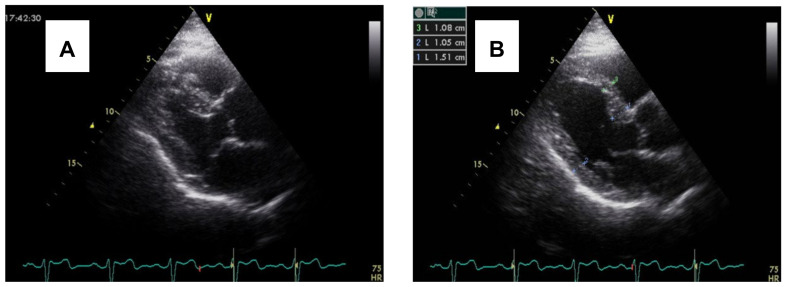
(A) Echocardiography shows a predominant regional LV septal base during end-diastole in a hypertensive patient with basal septal hypertrophy. (B) Protrusion of septal basal tissue into the LV outflow tract during end-systole in the same patient. (B) shows basal septal hypertrophy in a hypertensive patient.
